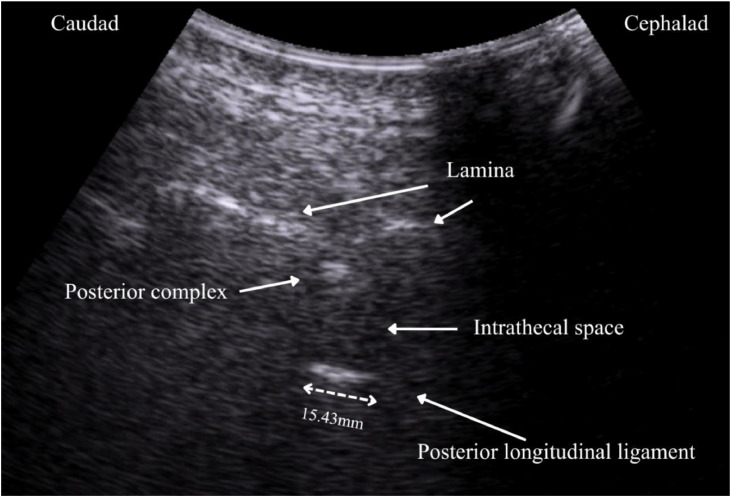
Structures of neuraxial ultrasound on paramedian sagittal oblique plane on L3‒L4 intervertebral space and PLL length along the largest axis. No patient identification, positioning, or level was present in the file name or image.
